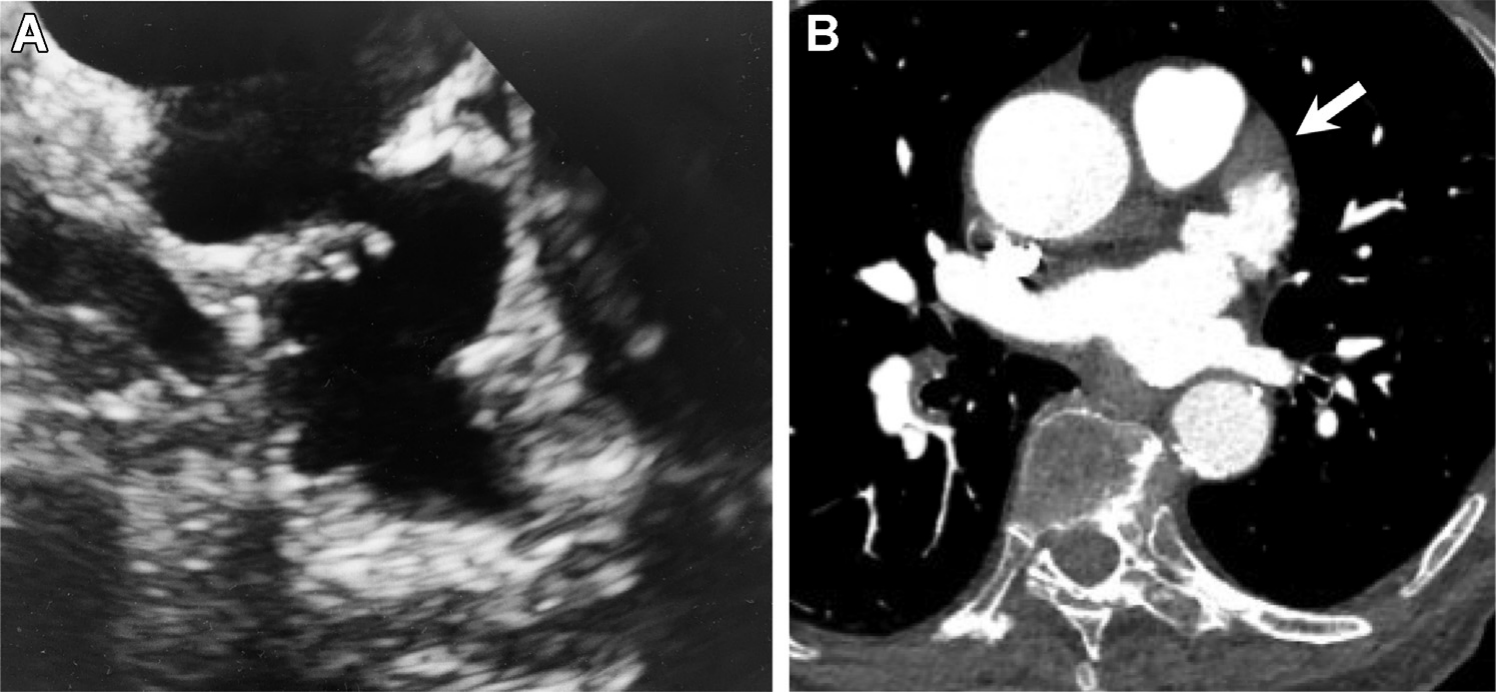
Preprocedure left atrial appendage (LAA) imaging. A: Transesophageal echocardiography (TEE) imaging at 135 degrees demonstrates serpiginous LAA anatomy. B: Similar anatomy is seen on a contrast-enhanced chest computed tomography scan. The filling defect at the LAA apex (*white arrow*) was secondary to low flow and poor contrast filling; no thrombus was seen on a subsequent intraprocedural TEE.
